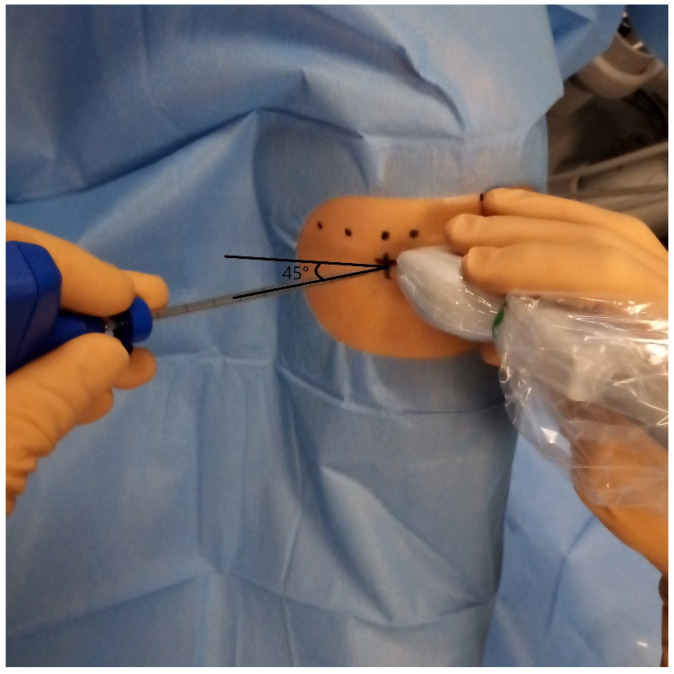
Ultrasound-guided puncture of the medial tibial endplate for bone marrow aspiration.
